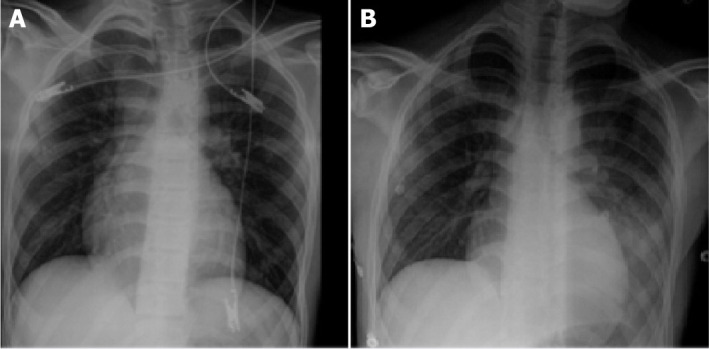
Patient’s initial PA chest radiograph (A) showing no pathological findings; patient’s 4^th^ day postero-anterior chest radiograph (B) showing heterogeneous density increment of lower zone of the left lung and blunting of the left costophrenic sinus.
